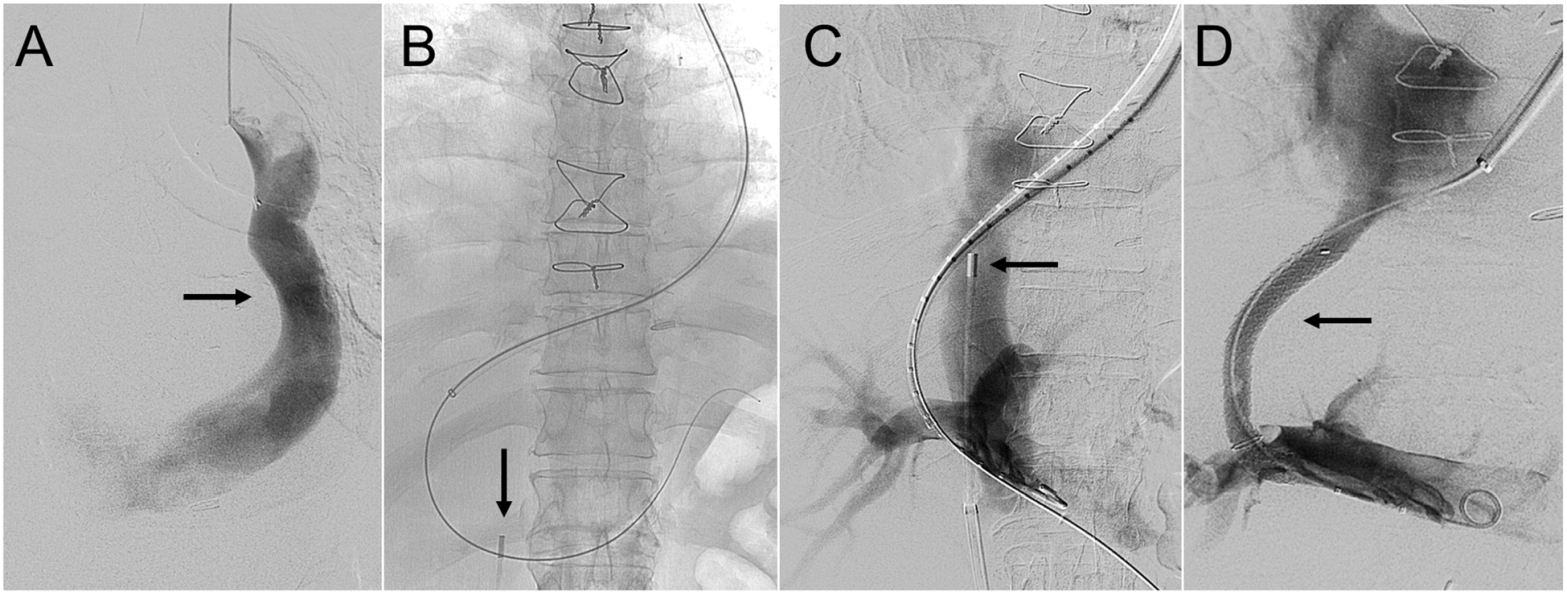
A middle-aged man with hepatic hydrothorax and isolated persistent left superior vena cava. a Left internal jugular venography demonstrating the isolated persistent left superior vena cava (solid arrow). b Access from the left internal jugular vein into the portal system via the left superior vena cava and coronary sinus using intravascular ultrasound-guidance (solid arrow). c The right portal vein was accessed with a Rösch-Uchida needle, and simultaneous portal and caval venography was performed. A perpendicular-projecting intravascular ultrasound probe (solid arrow) is shown. d An 8–10-mm × 8-cm (covered) / 2-cm (uncovered) Viatorr controlled expansion stent-graft (solid arrow) was deployed with resultant brisk in-line flow
